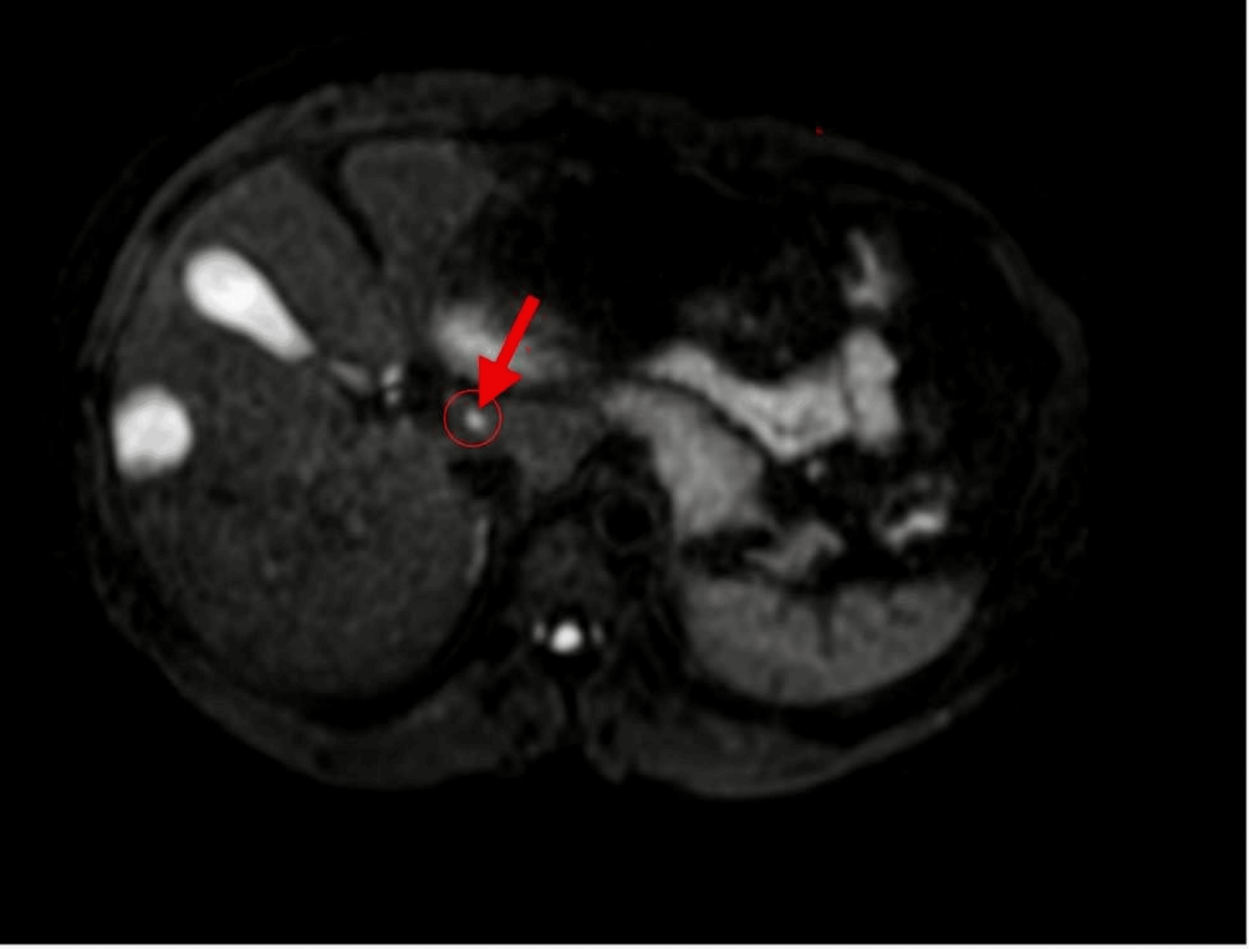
Abdominal MRI showing a lesion in the caudate lobe MRI: magnetic resonance imaging
